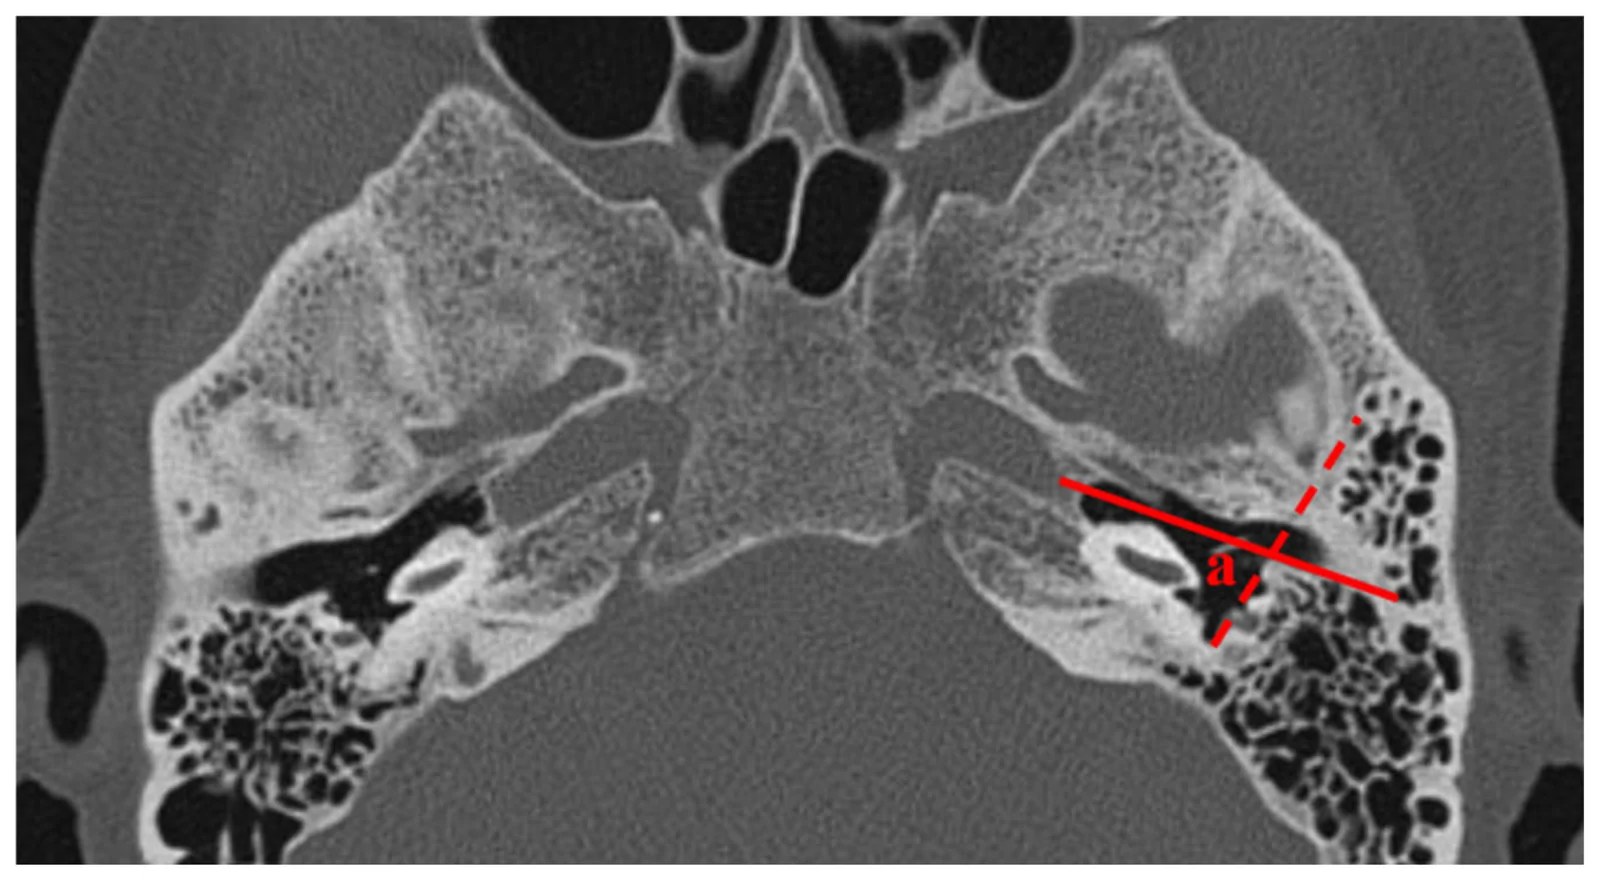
Axial view of high-resolution temporal bone computed tomography (CT) image revealing the scutum angle (a). The solid line represents the extension of the external auditory canal (EAC) wall, and the dotted line indicates the tympanic cavity wall. The angle formed between these two lines is the scutum angle.
